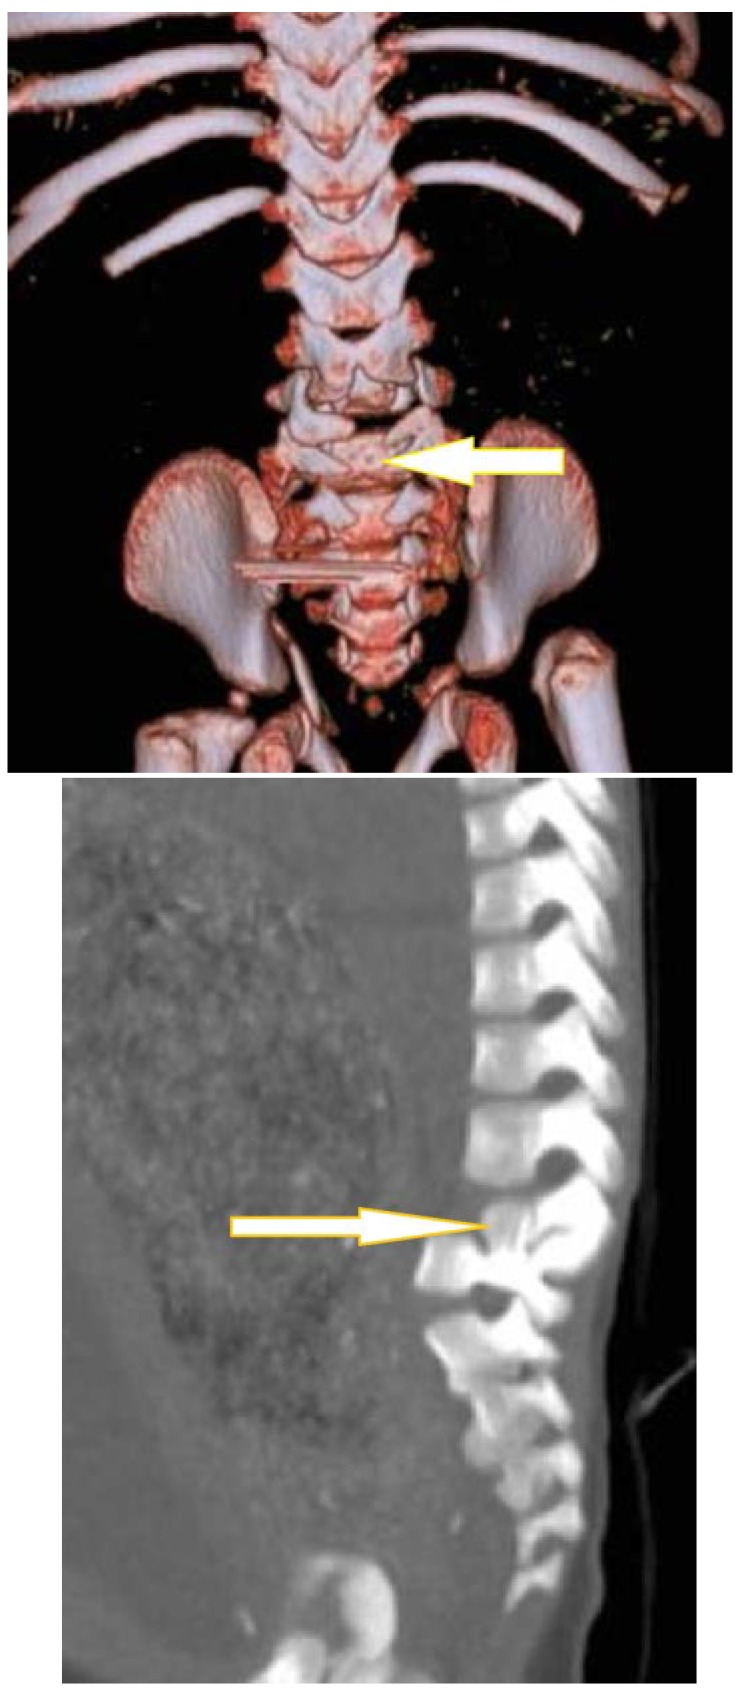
3D CT reconstruction in a 1-year-old patient with teratogenic spondylolysis: in coronal plane the image shows sacral spondyloschisis with left lamellar isthmic spondylolysis (left arrow) and the sagittal image highlights the posterior spondyloschisis with L3-L4 retrolisthesis (right arrow)
